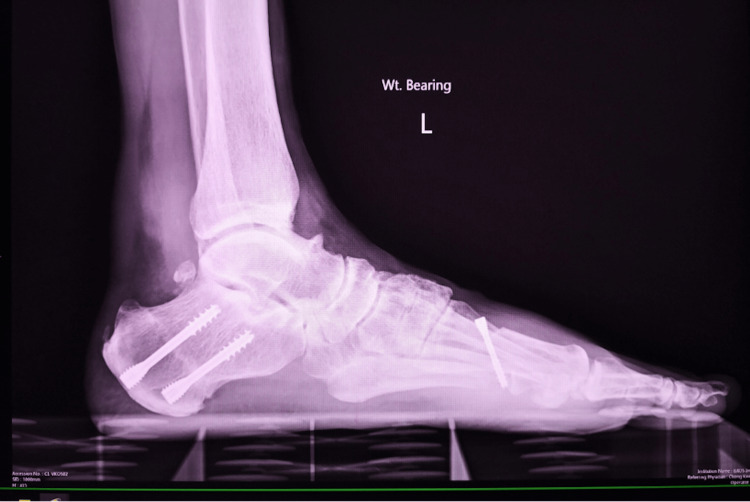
Post-operative weight-bearing radiographs of the patient’s left foot.
